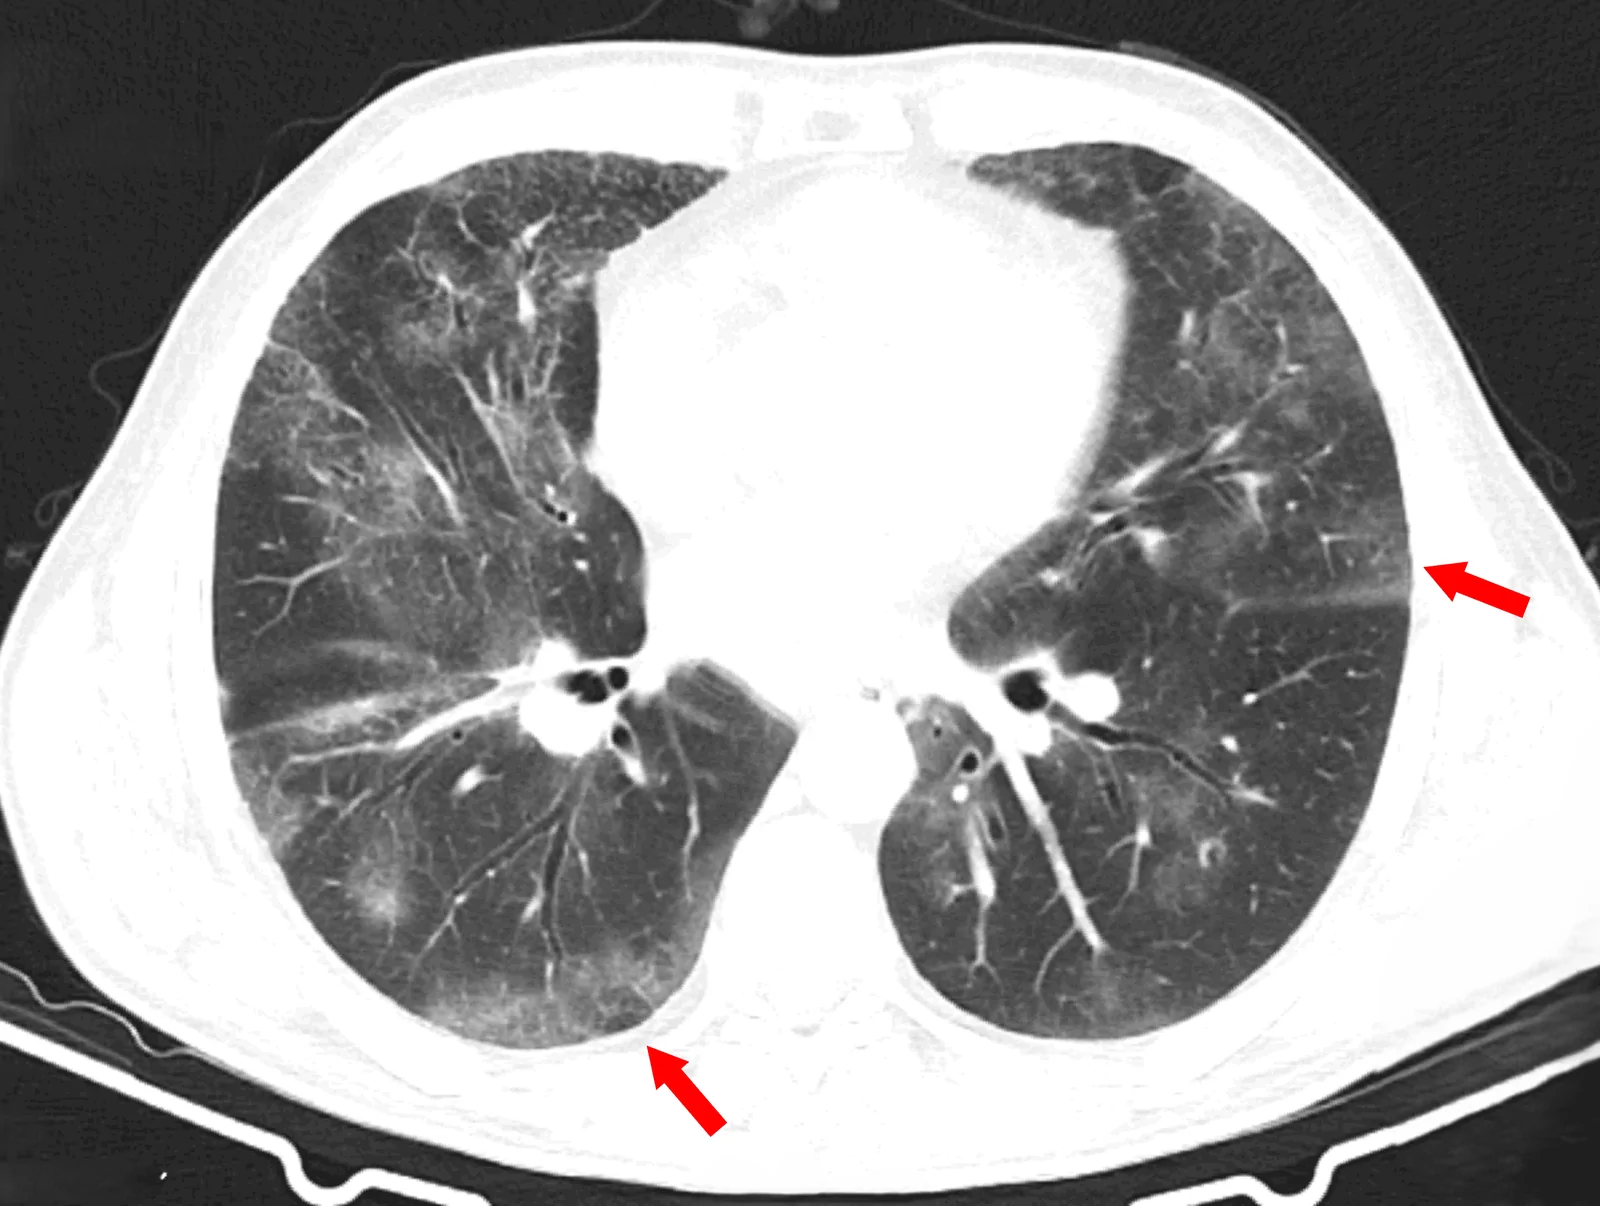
Axial CT image of the chest showing multifocal bilateral ground-glass opacities with a perilymphatic distribution (arrows).
